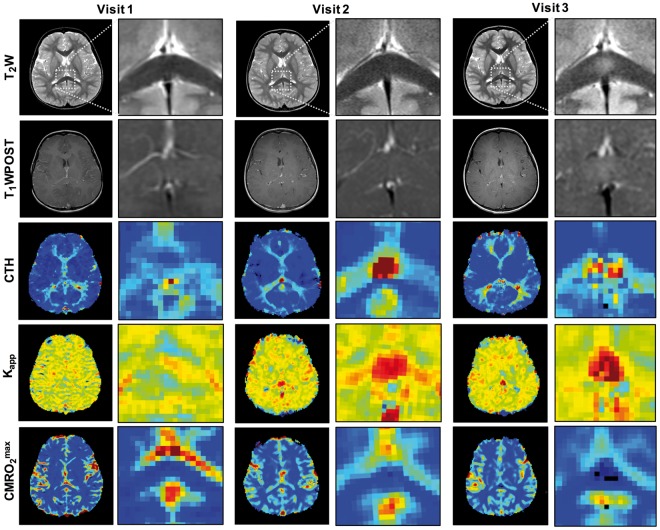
Microvascular perfusion in a patient converting to cerebral disease. Longitudinal sequential imaging in a young male hemizygote who converted to CALD. *Upper* two panels show structural T_2_-weighted (T_2_W) and T_1_-weighted post-contrast (T_1_WPOST) images. *Lower* panels show corresponding perfusion-based maps for each time point. Slightly elevated at the baseline visit, this patient developed a substantial peak in CTH and increased permeability (K_app_) within the splenium of the corpus callosum at the second visit 13 months later. While the T_2_-weighted- and T_1_-weighted post-contrast images showed no abnormalities at this time-point. Follow-up scan 6.5 months later revealed a corresponding T_2_-weighted-hyperintense region. Areas of increased CTH moved further outward from this lesion while blood–brain barrier permeability increased in the core. T_1_-weighted images show slight, hazy contrast enhancement. Low CMRO_2_^max^ coincides with appearance of the T_2_-weighted lesion. The subject underwent MRI serial screening due to confirmed hemizygote status, was successfully treated with HSCT and remained neurologically asymptomatic during the observation period.
